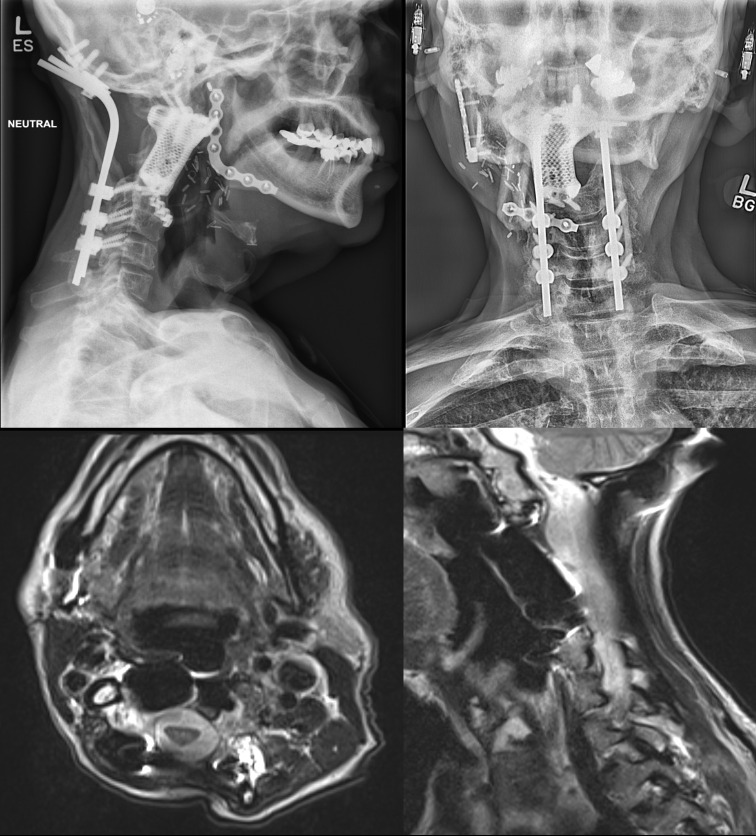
Postoperative imaging at two-year follow-up, including cervical radiographs that demonstrate maintained alignment and positioning of the 3DPI (upper images) and T2-weighted MRI that demonstrates the operative cavity without signs of recurrence (bottom images).
